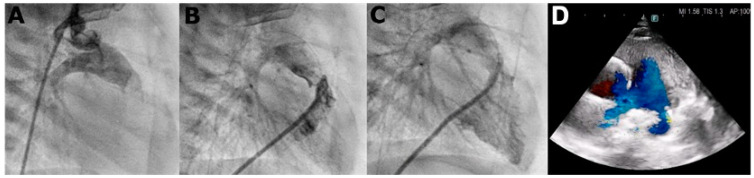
Angiography performed at the neonatal period showing: retrograde injection through the aorta showing pulmonary atresia (panel A), anterograde injection at the right ventricle outflow tract after perforation of the pulmonary valve (panel B), and final anterograde injection of the right ventricle after the final valvuloplasty (panel C). Echocardiography at 4 years-old showing the right ventricle outflow tract with the pulmonary arteries (panel D).
